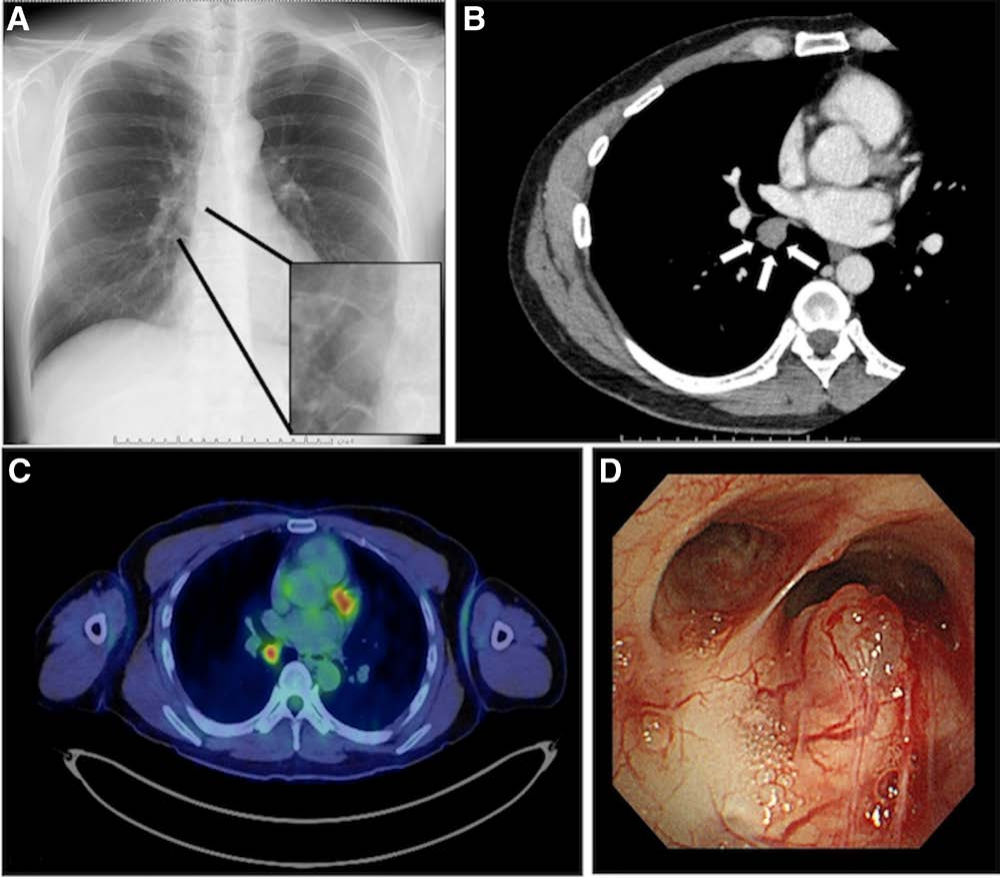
(a) A nodule is noted on the chest radiograph (black arrows). (b) A 17-mm-sized nodule, with a mild contrast effect, is noted on contrast-enhanced CT (white arrows). (c) 18F-fluorodeoxyglucose (FDG) positron emission tomography/computed tomography (PET-CT) reveals abnormal FDG accumulation in the nodule, with a maximum standardized uptake value of 4.07. (d) Bronchoscopy reveals a non-pulsating submucosal nodule, with proliferating capillary vessels, on the mucosal surface of the proximal end of the right intermediate bronchus.
